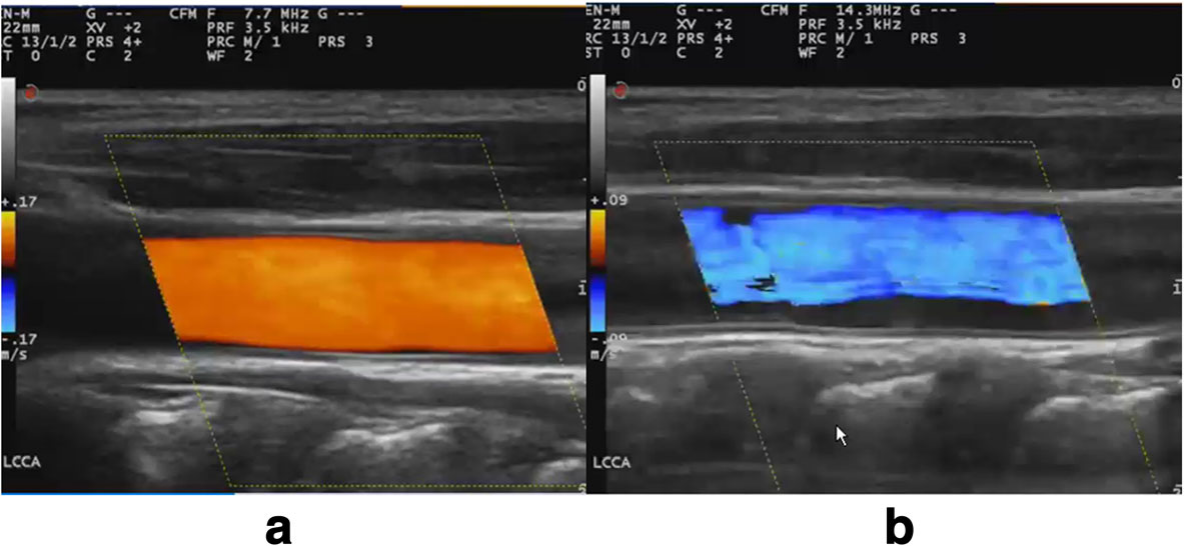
A lower Doppler frequency allows more penetration but also a lower resolution. A higher Doppler frequency gives a more detailed image of the vessels at the expense of penetration. *Left*: carotid artery with a Doppler frequency of 7.7 MHz. *Right*: carotid artery with a Doppler frequency of 14.3 MHz; this cannot penetrate well enough to give optimal flow information
